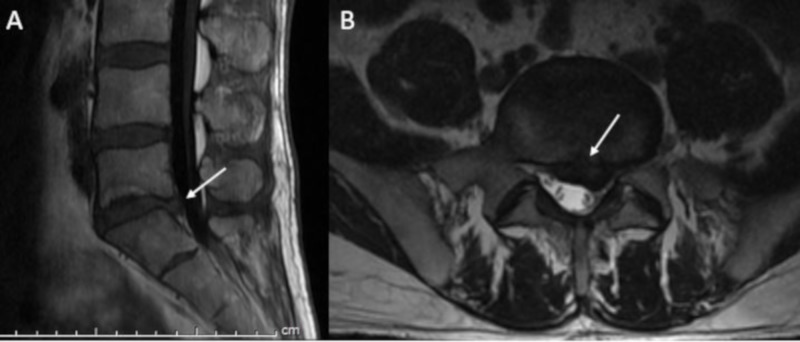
Case 2 diagnostic images. (A) Sagittal T1 FLAIR and (B) axial T2 weighted images of the lumbar spine demonstrate a large, central to left-sided disk herniation with extruded disk compressing the left S1 nerve root. Arrows highlight areas of interest.
